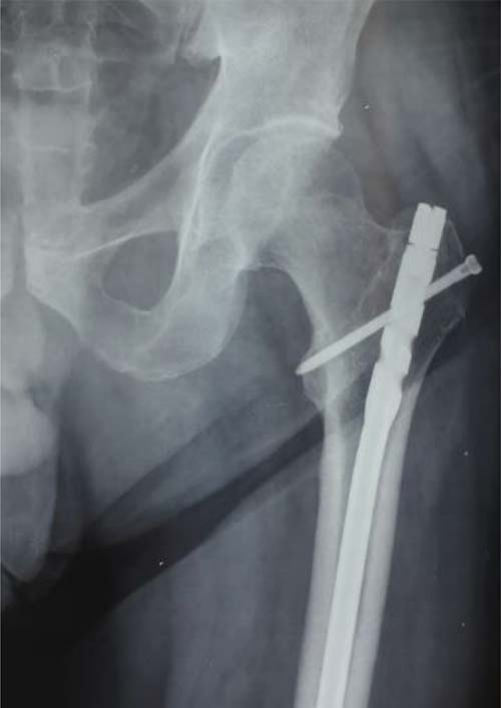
Anteroposterior radiograph showing the tip of the intramedullary nail right at the level of the greater trochanter.
